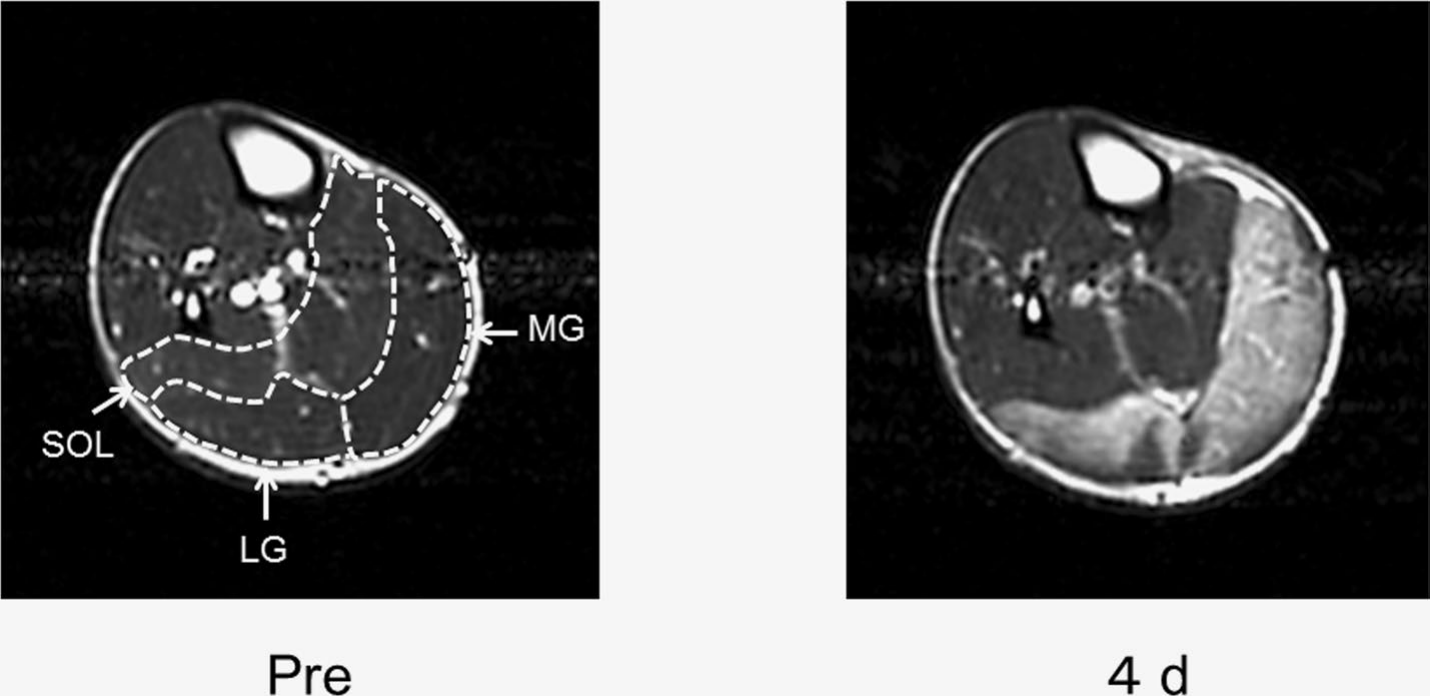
Axial T2-weighted images (repetition time = 2,500 ms, echo time = 80 ms) of the right leg before and at day 4 post-exercise. Increased signal intensity was more prominent in the MG on post-exercise T2-weighted image (MG: medial gastrocnemius; LG: lateral gastrocnemius; and SOL: soleus). This increased signal intensity reflects increased fluid content within the MG. In particular, the size of the MG apparently increased at day 4 after exercise, likely due to the edema formation.
